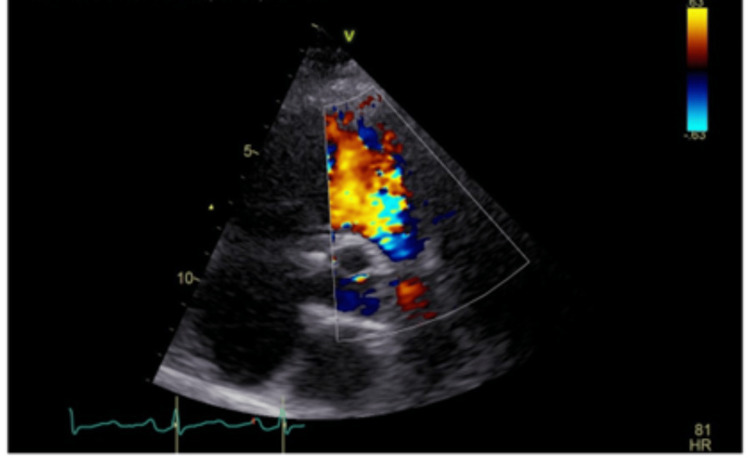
Severe pulmonary regurgitation on echocardiogram.
